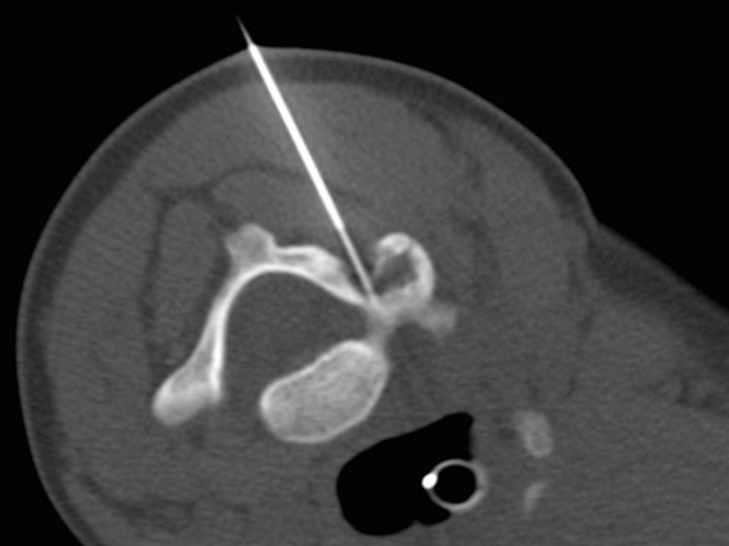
A 15-month-old boy suspected to have spondylodiscitis. CT-guided needle aspiration was performed for analysis
